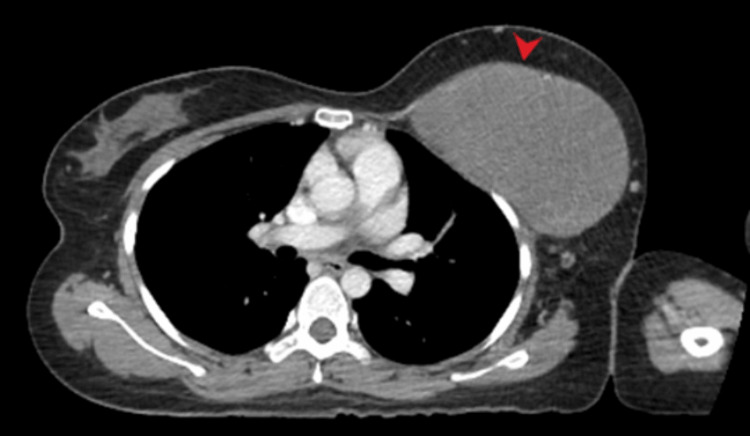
Axial post contrast CT chest at the level of upper chest shows a well-circumscribed soft tissue density lesion inseparable from underlying chest wall muscles (annotated by red arrow head).
